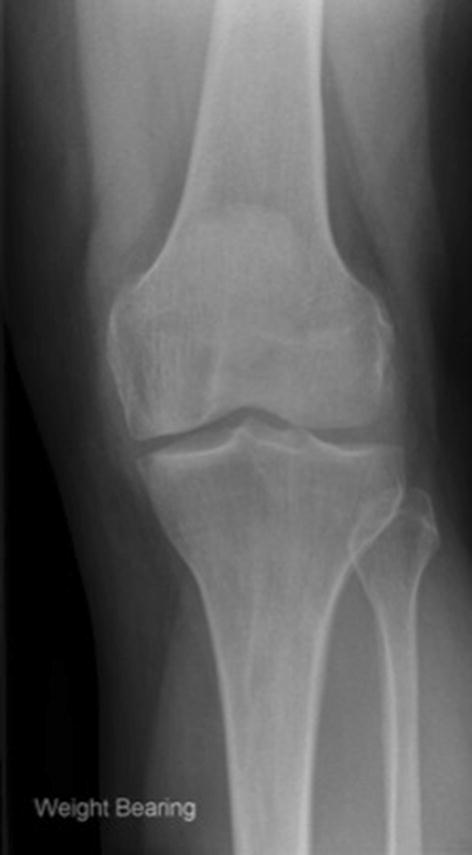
A preoperative weight-bearing anteroposterior radiograph demonstrating preserved medial joint space. This represents partial thickness cartilage loss and is a contraindication to unicompartmental knee replacement (UKR); UKR performed in these patients have a higher incidence of reoperation, revision and persistent post-operative pain
